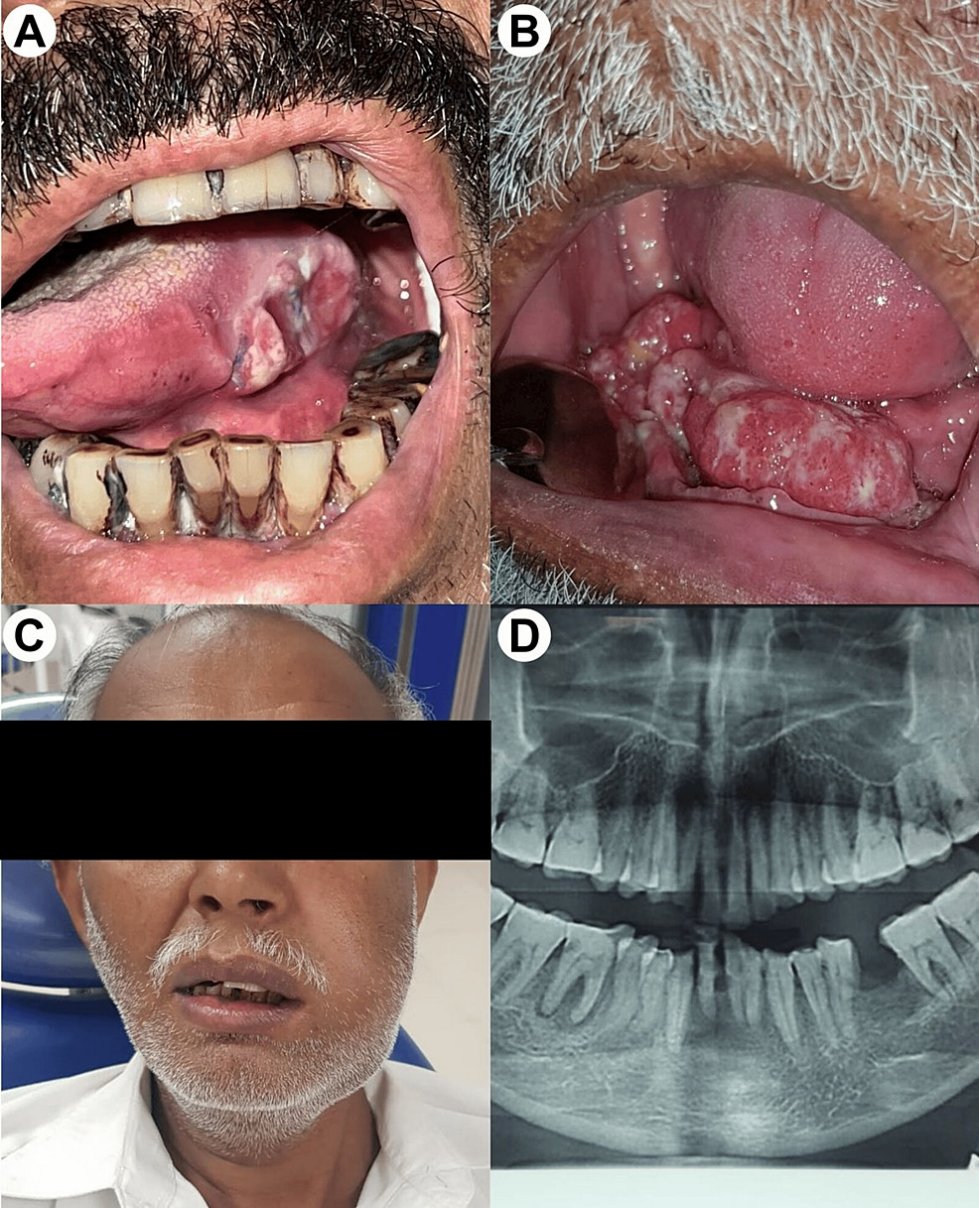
Clinical and radiographic presentation of OSCC. (A) A chronic paan (betel quid) chewer with an ulcerated mass involving the posterior-lateral border, base of the tongue, and floor of the mouth. (B) A large exophytic mass involving the mandibular edentulous ridge with surface ulceration. (C) A hard buccal mucosal and alveolar ridge mass, clinically visible as extra-oral swelling. (D) An OPG (orthopantomogram) of the same patient showing radiolucency and erosion of alveolar bone at the extracted socket area.
